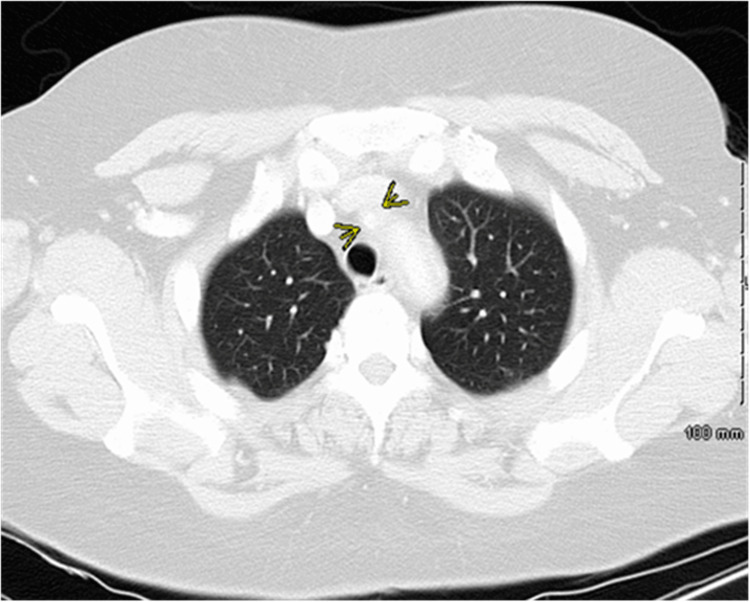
Computed tomography angiography of chest, axial view The yellow arrow indicates mural thickening that extends into the proximal aortic arch branching vessels.
